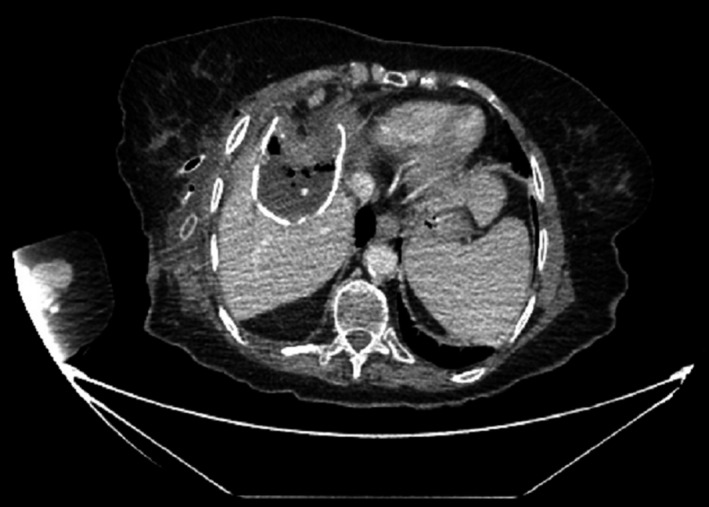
CT scan of the abdomen, showing a calcified hydatid liver cyst, with a fistulous track extending to the thoracic skin level.
